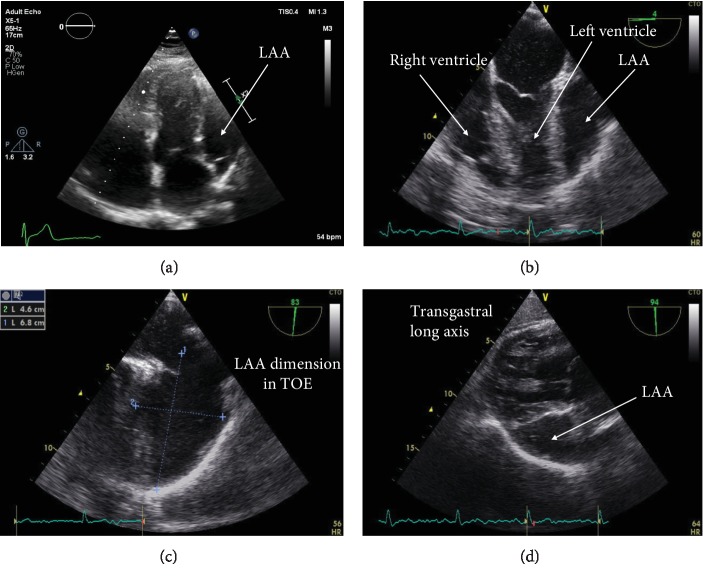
Echocardiographic views of the LAA.
